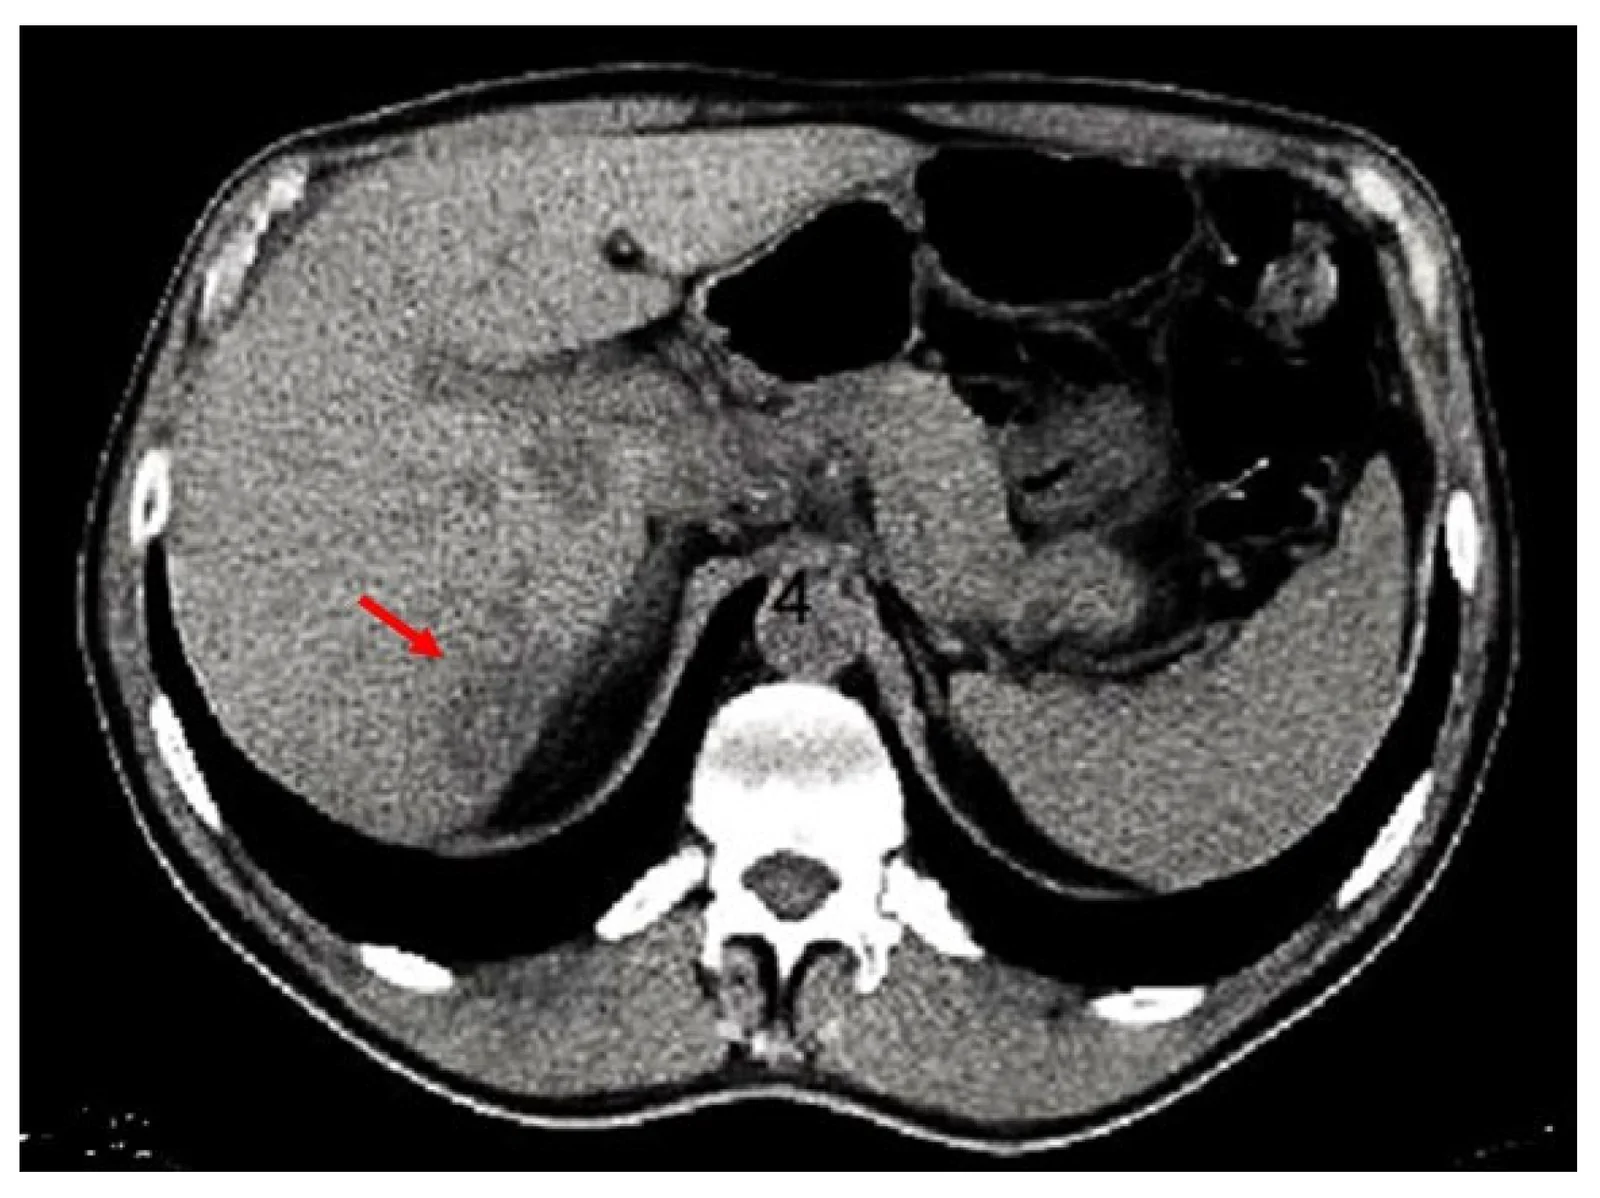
The larger liver lesion in segment 8 appears to have completely resolved, while the other lesion showed a significant reduction in size (red arrow) following effective antiparasitic treatment, as demonstrated by the CT performed 6 months later.
